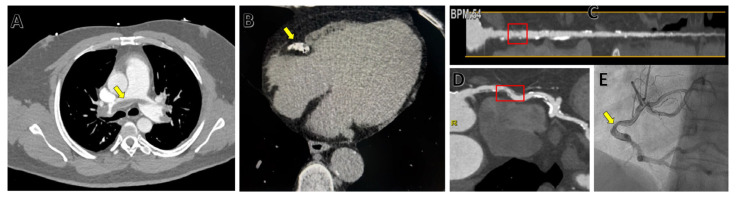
Examples of CT findings in COVID-19 patients. (A) Bilateral pulmonary artery embolism (yellow arrow) on an axial plane. (B) Large coronary calcified plaque on the right coronary artery (yellow arrow). (C) Straightened multiplanar reconstruction (MPR) of the right coronary artery showing moderate stenosis (60%–red box) in coronary CT angiography. (D) Curved multiplanar reconstruction (MPR) of the right coronary artery showing moderate stenosis (60%–red box) in coronary CT angiography. (E) Invasive coronary angiography confirming moderate stenosis (yellow arrow) of the right coronary artery. ((B–E) are for the same COVID-19 patient with chest pain, who also presented anomalous origin in the left anterior descending and circumflex arteries).
